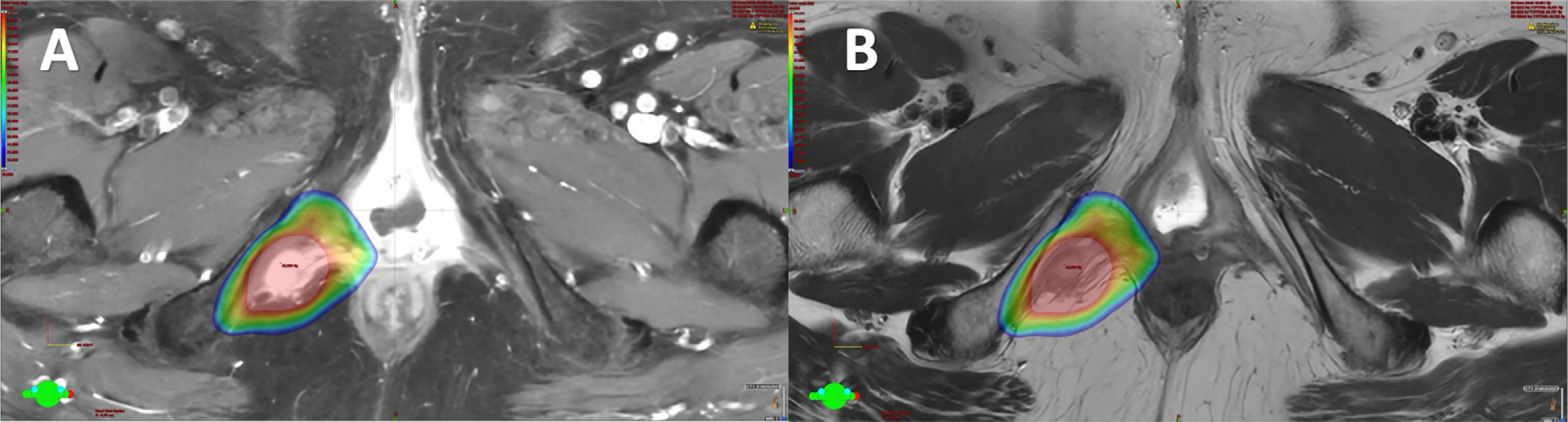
SABR dose displayed on co-registered MRI scan in August 2023 (A, T1w+C MRI scan; B, MRI T2w scan) Radiotherapy software-generated 360 degrees images displayed in green Fig. to orient the imaging plane.
